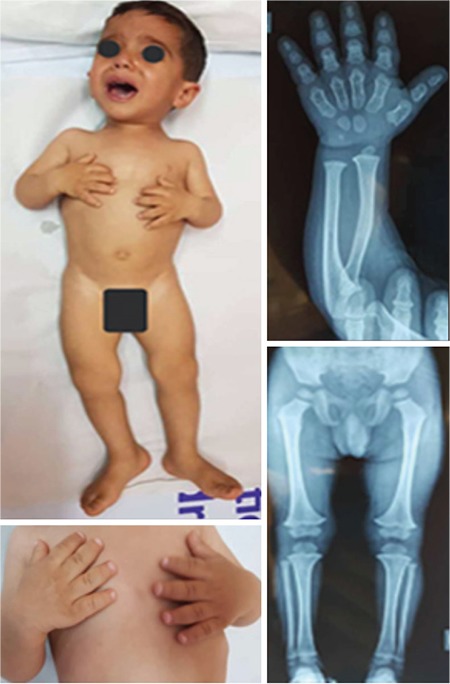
Clinical characteristics and radiographic features of the patient. Frontal bossing, low set ears and wrist joint hyperflexibility as well as short and broad fingers of the hand with small nails are noteworthy. Radiographic findings showed radial bowing with posterior dislocation, short lower end of the ulna as compared to the radius, and broad metacarps with wide phalanges
